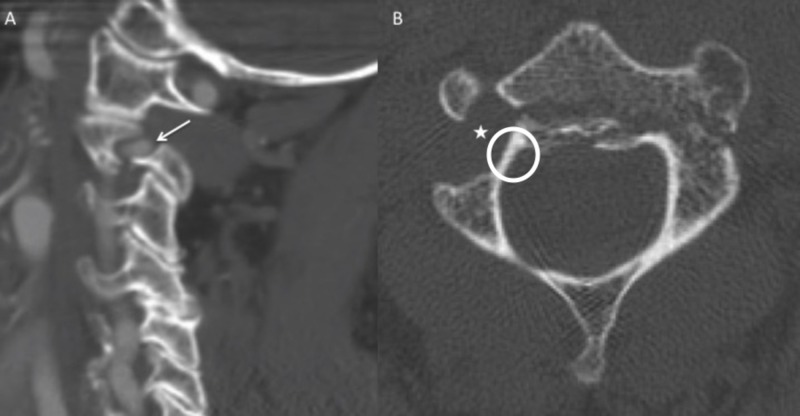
Computed tomographic angiogram (CTA) images. (A) Sagittal CTA images showing the large right dominant vertebral artery with congenital dehiscence of the superior portion of the foramen (arrow). This was an important consideration in exposure of the right C1 lateral mass to avoid iatrogenic vertebral artery injury. (B)  Noncontrasted axial CT showing a large vertebral foramen on the right side (star) with a congenitally small C2 pedicle (circle). A horizontal fracture through the posterior portion of the vertebral body is also seen.
